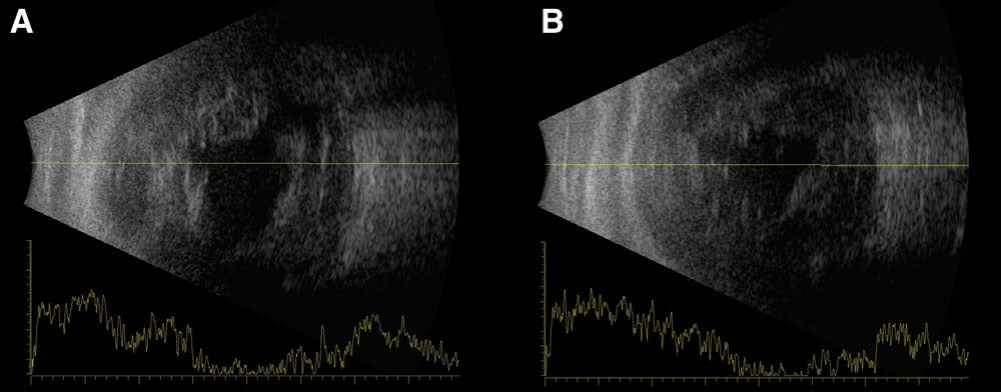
Preoperative B-scan ultrasonography of the right eye. (A) Horizontal and (B) vertical scans showing choroidal detachment and vitreous hemorrhage.
